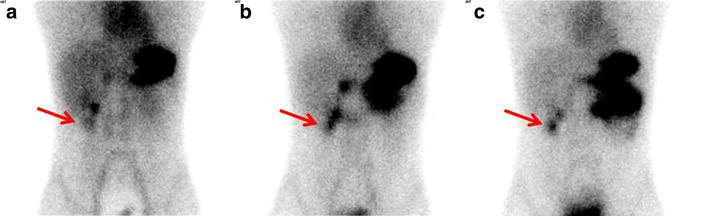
5 min (a), 20 min (b) and 60 min (c) after administering 1.5 mCi of Technetium-99 m pertechnetate intravenously, planar scintigraphy was performed respectively. Images showed two focal concentrations under the liver at the right lower quadrant of abdomen region
